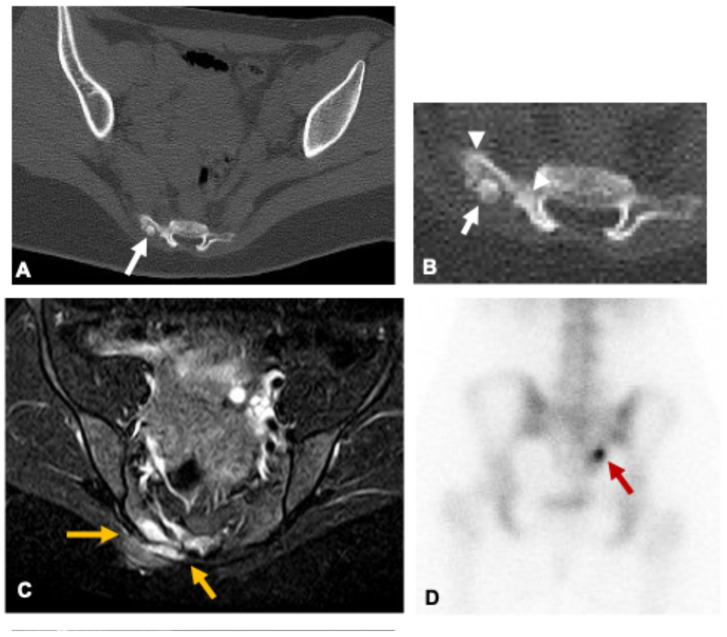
Osteoid osteoma in a 16-year-old girl. Axial CT images (A,B) show a small osteolytic lesion in the right lateral aspect of S3 with a central calcified nidus (white arrows) and surrounding sclerosis (arrowheads), findings typical of mineralized osteoid osteoma. On axial fat-saturated T2-weighted MRI (C), the nidus is not clearly visualized; instead, prominent bone marrow and adjacent soft-tissue edema are noted (yellow arrows). Bone scintigraphy (D) demonstrates focal radiotracer uptake (red arrow) consistent with high osteoblastic activity. The patient presented with persistent nocturnal sacral pain relieved by NSAIDs, associated with localized tenderness and occasional radiation to the right gluteal region.
